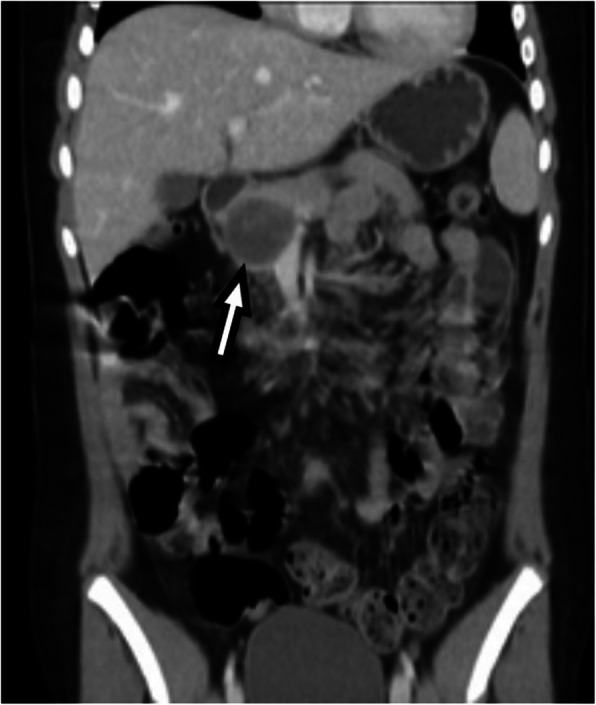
CT formation at the border of the head and body of the pancreas is a heterogeneous hypodense formation, with uneven internal contours, 37 × 38 × 35 mm in size, with a density of up to 40 units. HU, with contrast enhancement of blood flow, is not visualized
